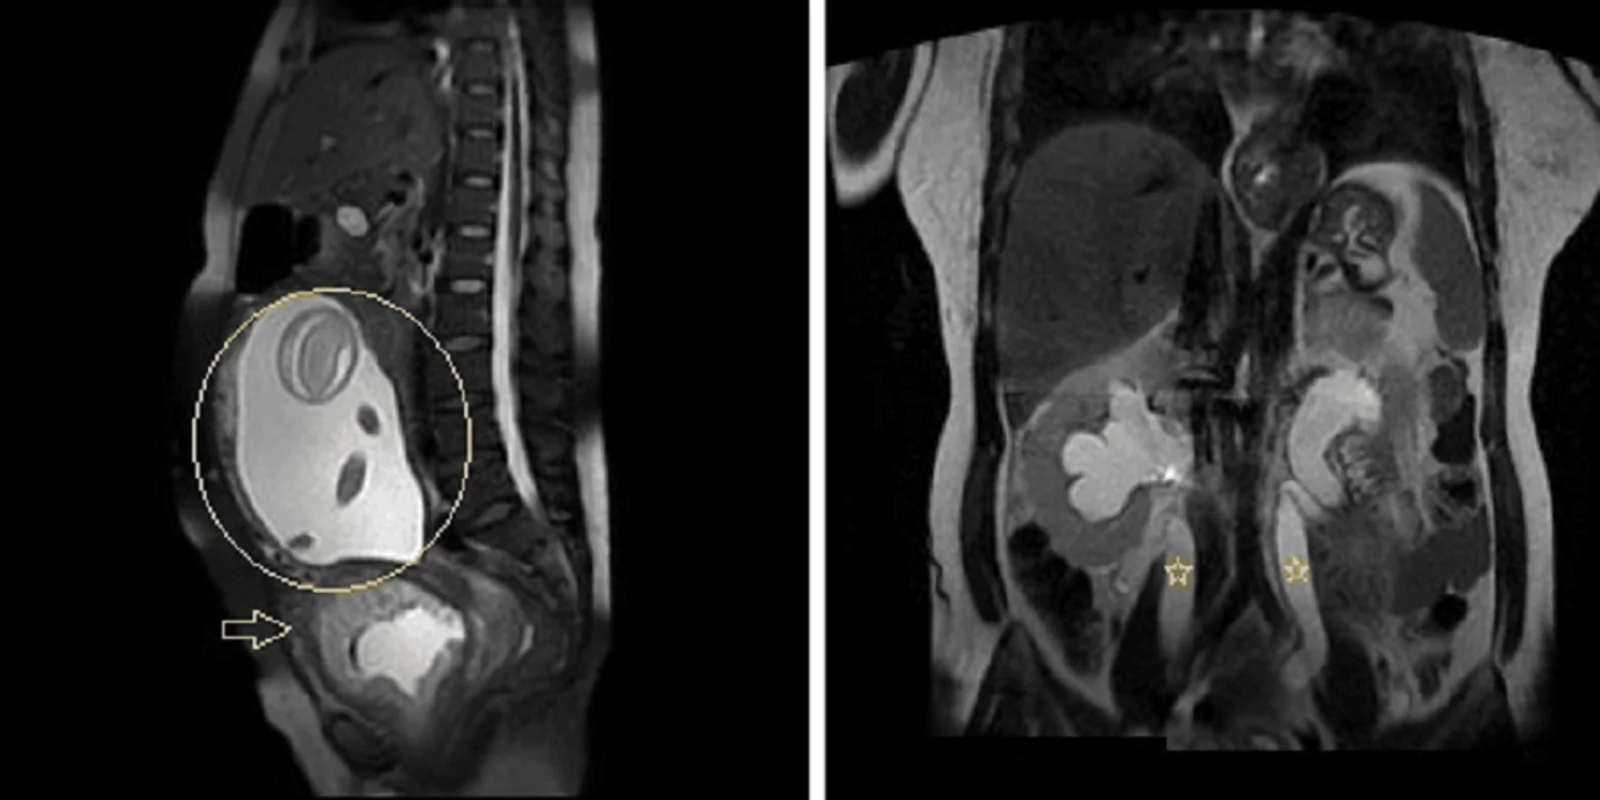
Pelvic MRI during pregnancy showing a circumferential invasive bladder tumor (arrows) with bilateral hydroureteronephrosis (stars) and the adjacent gravid uterus (circle).
